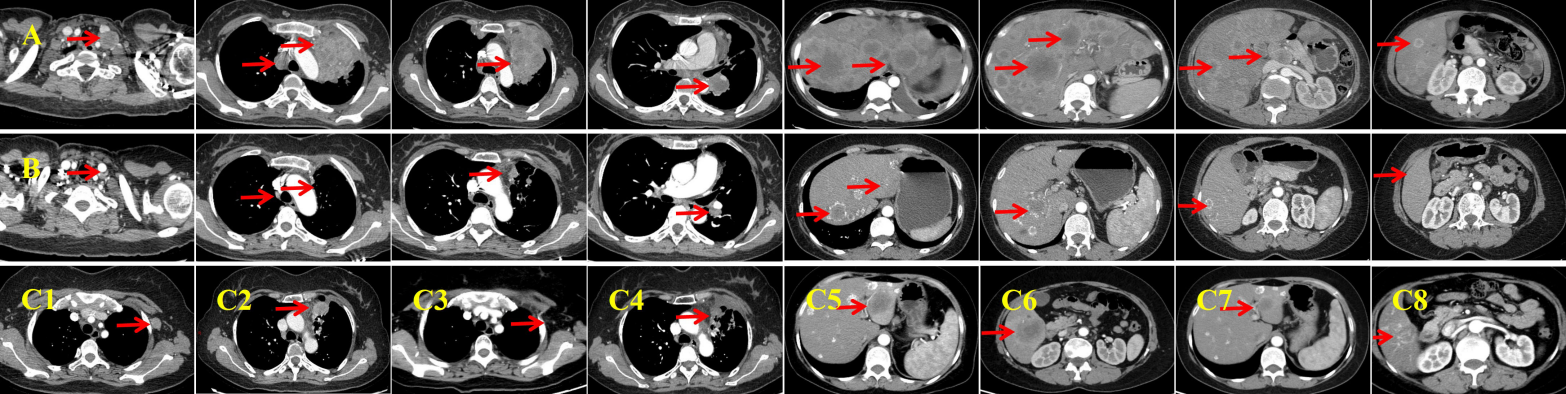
Baseline imaging and first-line therapy assessments. (A) Baseline contrast-enhanced computed tomography (CT) performed on January 14, 2021 reveals a left lung mass measuring approximately 55 × 80 mm, with associated lymphadenopathy and liver metastases. The lesions exhibit marked central necrosis and heterogeneous enhancement. (B) After six cycles of first-line therapy, an enhanced CT on July 29, 2021 demonstrates significant shrinkage of all lesions and the appearance of calcification in the liver metastases. (C, C1, C2) Follow-up enhanced CT on October 26, 2021 indicates progression of the primary lung lesion and the emergence of a new, enlarged lymph node in the left axilla. (C3, C4) Post-radiotherapy enhanced CT (January 22, 2022) shows shrinkage of the primary lesion and near-complete resolution of the left axillary lymph node. The response is classified as a partial response (PR). (C5, C6) Enhanced CT performed on July 28, 2022 reveals new metastatic lesions in the liver, indicating progressive disease. (C7, C8) Following three cycles of resumed first-line systemic therapy combined with local interventional treatment, an enhanced CT on November 2, 2022 shows significant reduction of liver metastases. The imaging response is again classified as PR.
